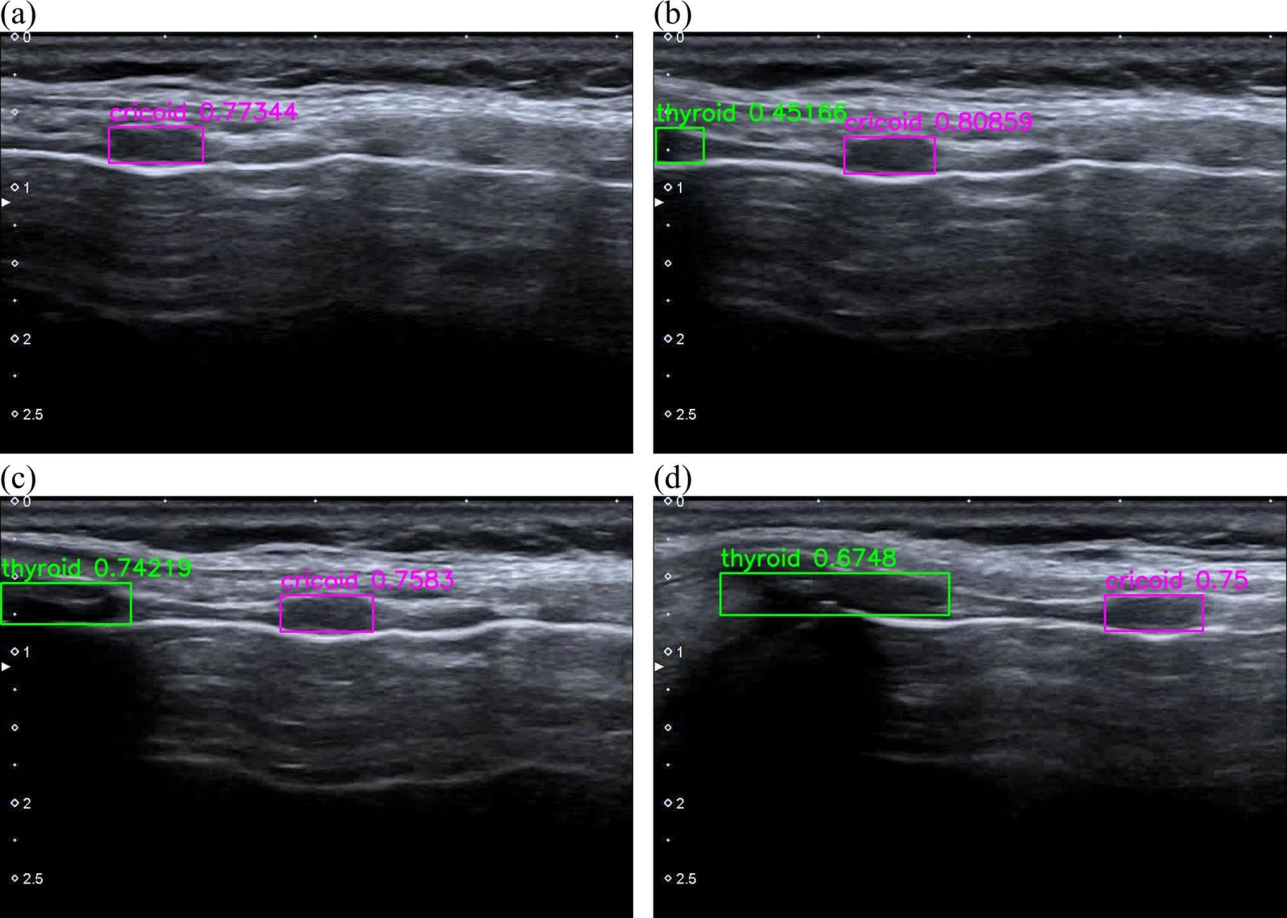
Representative frames demonstrating how YOLOv5s CC/TC prediction model outputs the predicted bounding boxes. The complete video clip can be watched in Video A.2. The image sequence from (a) to (d) represents the images the sonographers see when they move the transducer from the suprasternal notch cephalad. The pink rectangles indicate the predicted bounding box of the cricoid cartilage and the green rectangles refer to the predicted bounding box of the thyroid cartilage. The output probabilities of the predicted bounding boxes are annotated along with the predicted bounding boxes. Please refer to Video A.2 for the complete video clip
